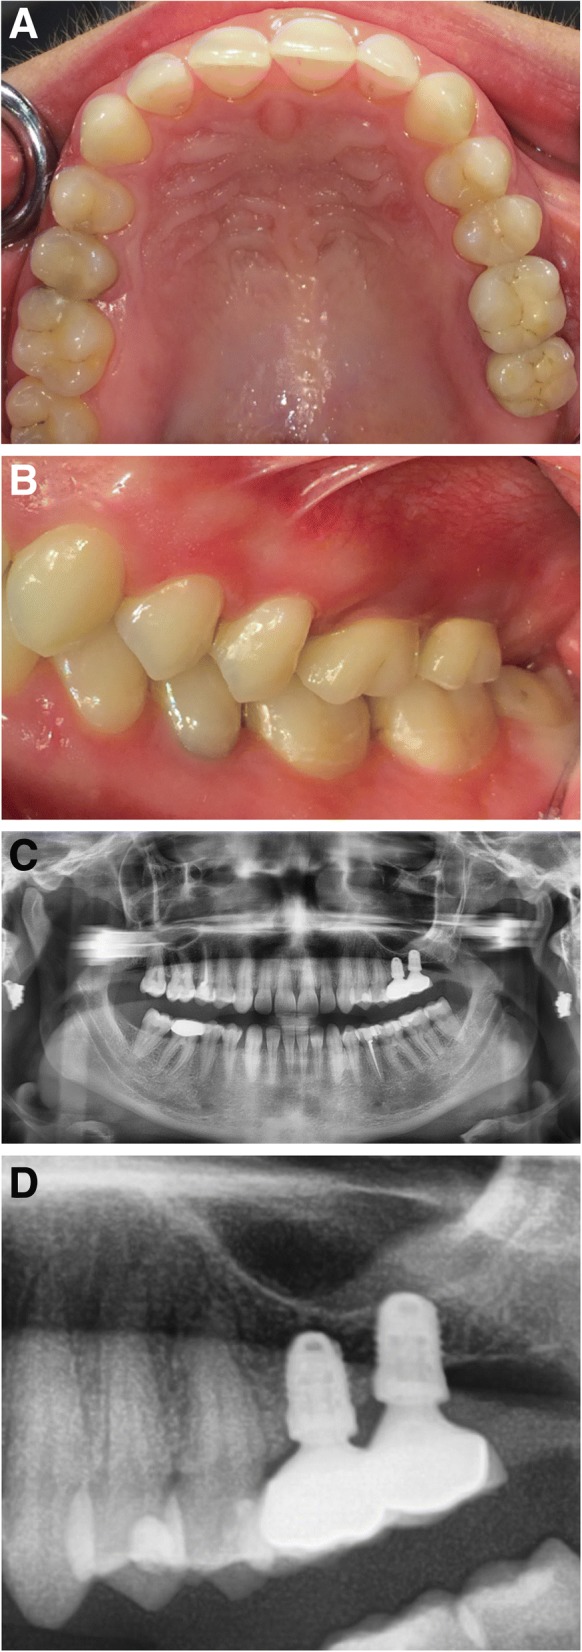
The clinical images of patient 4, with implant-supported single crowns in regions 26 and 27. No signs of a peri-implant infection, mucositis, peri-implantitis, or marginal bone loss were detected. a Occlusal view. b Left-side view. c Orthopantogram. d Close-up radiographic view
